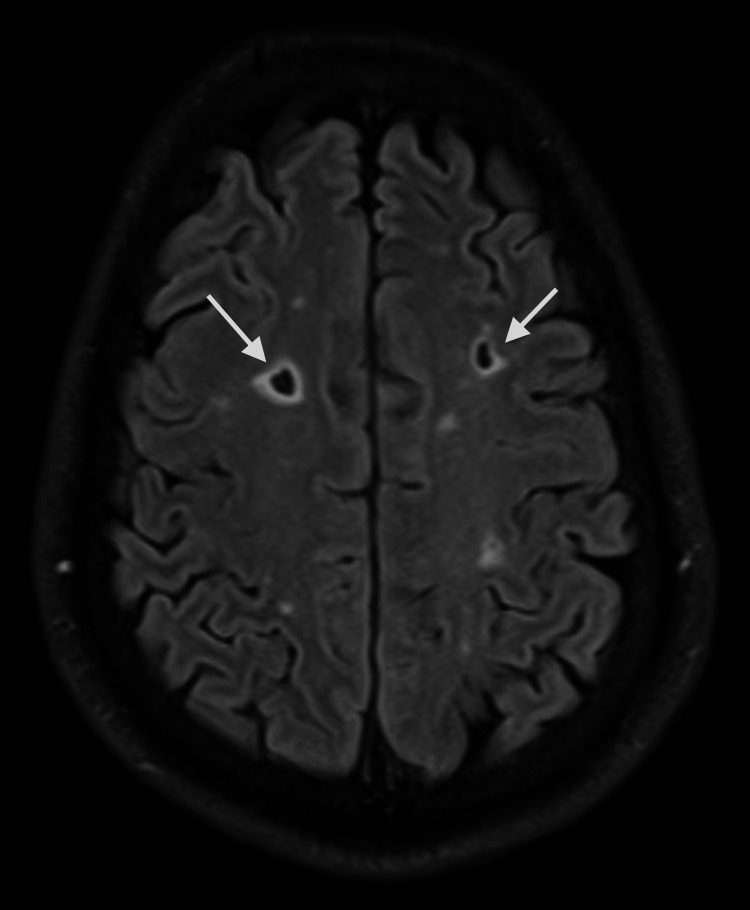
Brain MRI, FLAIR, axial view
